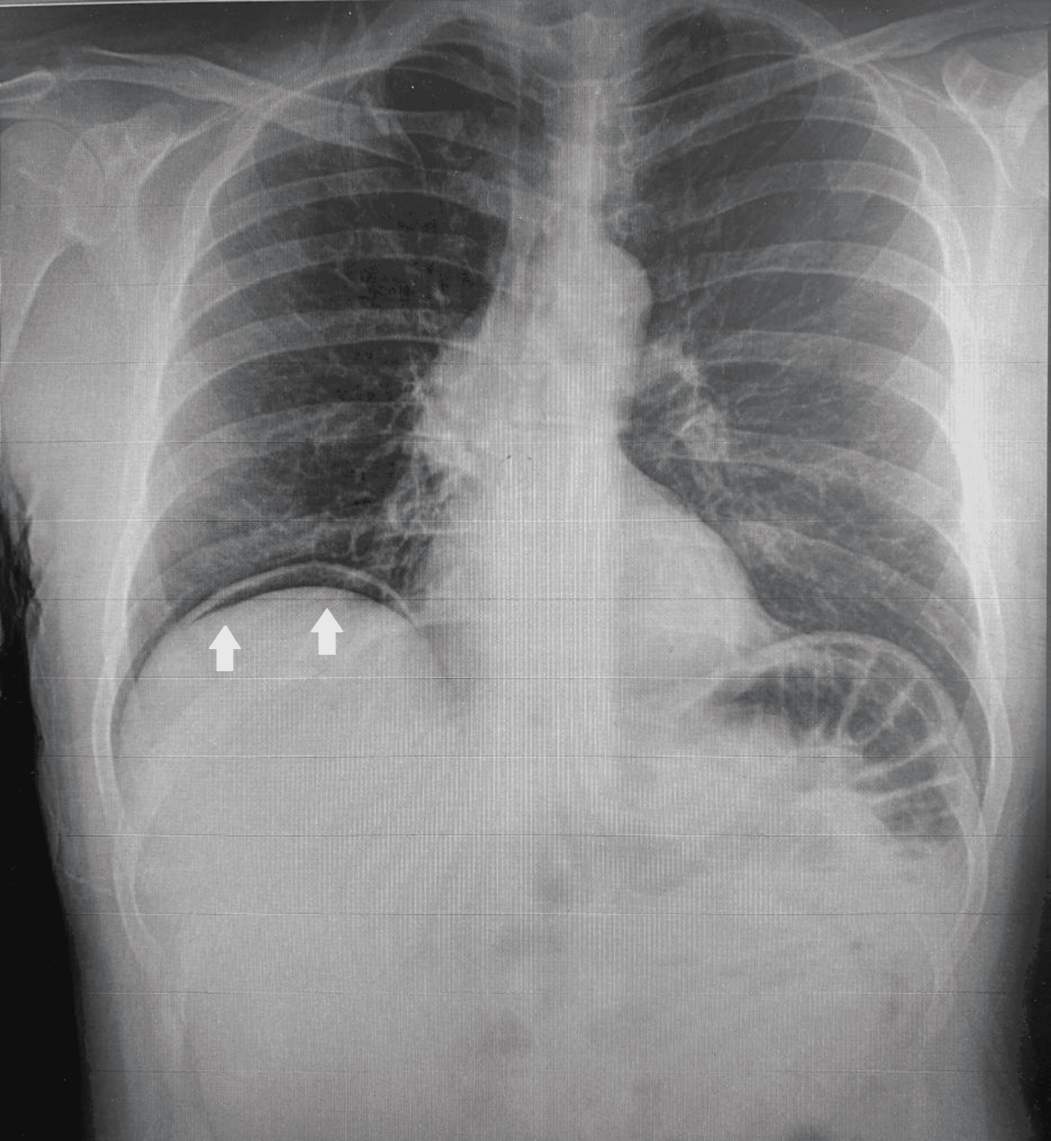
Chest X-ray showing gas under the diaphragm.
